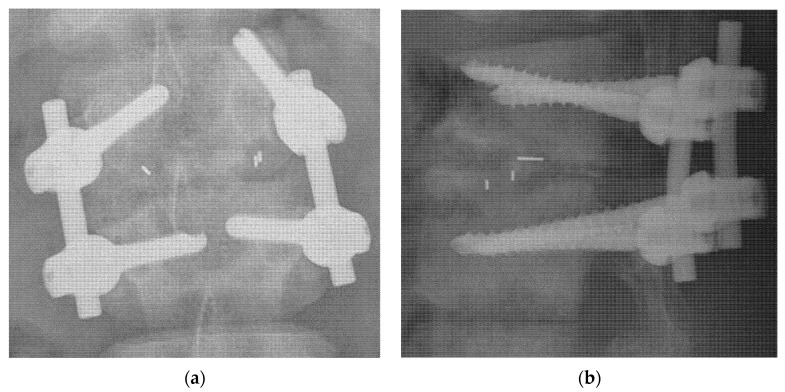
Radiographs after L1–2 TLIF surgery of a 64-year-old woman with ESRD. (a) Pedicle screw loosening on the left side of the L1. (b) Grade 4 fusion status and grade 3 cage subsidence with bone resorption around cage. TLIF, transforaminal lumbar interbody fusion; ESRD, end-stage renal disease.
